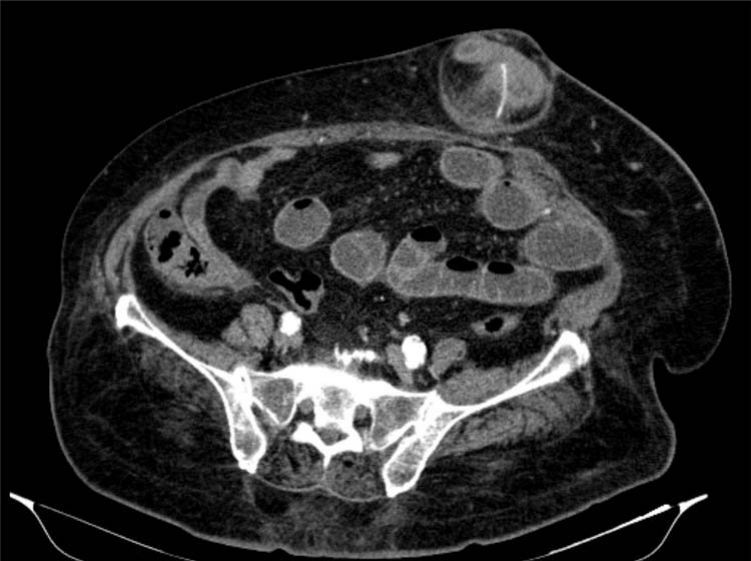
Abdominal CT: image of high-density and filiform morphology compatible with FB.
